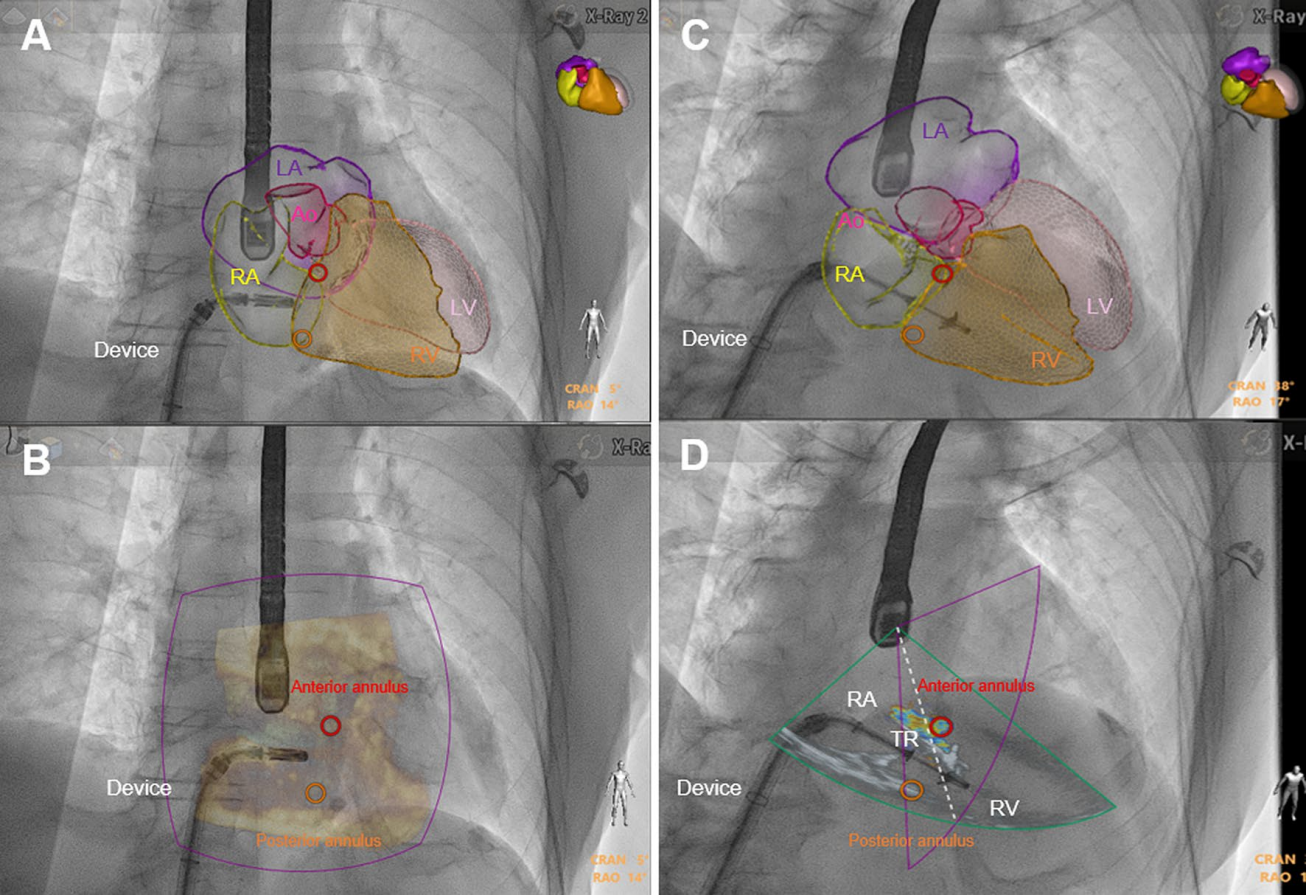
Real-time fusion imaging for guiding TTVR. Fusion of echocardiographic and X-ray images provides the superimposed echo-derived heart cavities and anatomical landmarks (orange marker—posterior anulus, red marker—anterior anulus) onto live X-ray fluoroscopy. A, B Real-time fusion imaging assisted navigation of the TTVR device through the right atrium pointing to the tricuspid annulus. C Safe device passage into the right ventricle. D The real-time overlay of the colour doppler onto fluoroscopy helps navigating the clip to valvular regurgitation jets. *RA* right atrium, *LA* left atrium, *RV* right ventricle, *LV* left ventricle, *Ao* aortae, *RVOT* right ventricular outflow tract, *TR* tricuspid regurgitation
